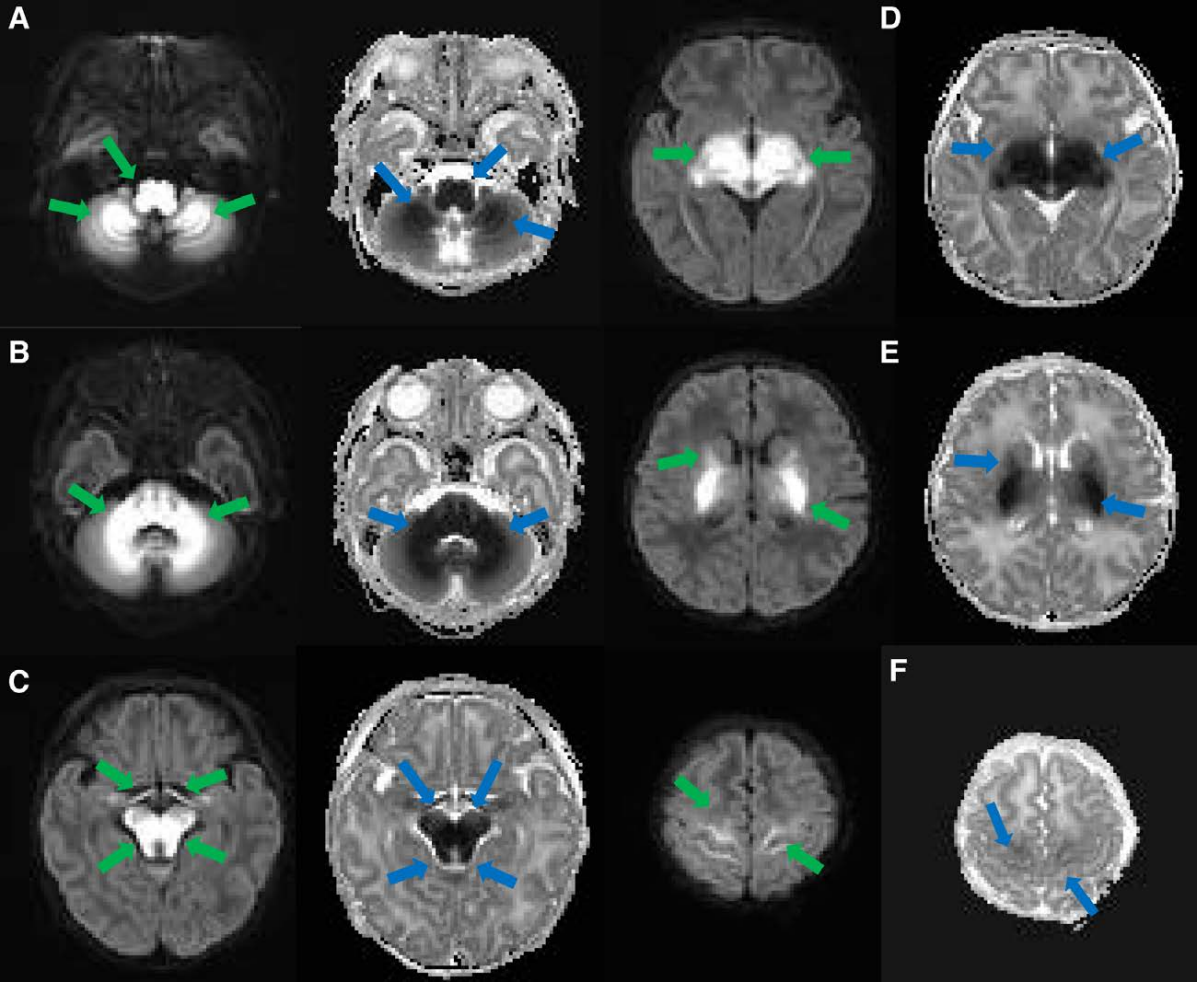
Axial DWI and ADC images of an 8-day-old female with MSUD. (a‐f) DWI and corresponding ADC images showing restricted diffusion in the brainstem, bilateral cerebellar hemispheres, basal ganglia, thalamus precentral gyrus and postcentral gyrus; DWI showed high signal intensity (green arrow), ADC showed low signal intensity (blue arrow), myelin or cytotoxic edema. ADC = apparent diffusion coefficient, DWI = diffusion-weighted imaging, MSUD = maple syrup urine disease.
